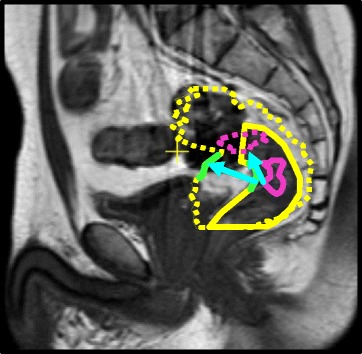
Delineations of the rectal wall (yellow), nearby rectal wall (green) and tumor (purple) on a sagittal MRI scan. The dashed delineations are overlaid from a different treatment day. Note the difference in amount of displacement and direction, between tumor and nearby rectal wall (cyan arrows)
